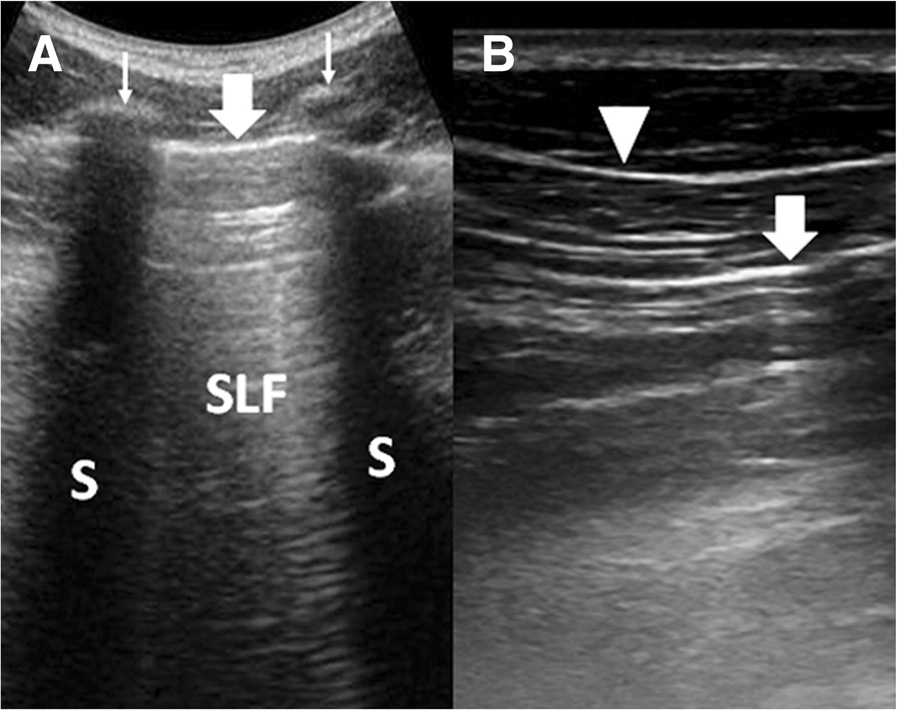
Comparing two scanning planes in LUS. a LUS performed in the longitudinal or cranio-caudal plane showed ribs (*thin arrows*) and their acoustic shadows (*S*). Just below the level of the ribs is the pleural line (*thick arrow*) and the sonographic lung field (SLF). b Subcutaneous tissue lines (*arrowhead*) could be mistaken for the pleural line (*arrow*) when LUS is performed in a transverse plane, without the guidance of the rib structure
